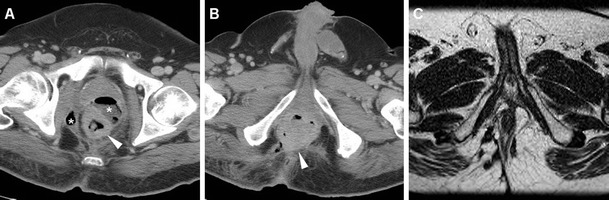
A 53-year-old man with purulent drainage and clinical diagnosis of perianal inflammation. Axial images (b,c) from urgent contrast-enhanced MDCT show solid circumferential thickening of the anal canal (*arrowheads*) associated with abscess collections with mixed gas-fluid content (*) and fistulas crossing the ischioanal space. Surgical examination under anaesthesia including biopsies revealed ulcerated SCAC with superinfection. After surgical drainage and subsequent chemo-radiotherapy, MRI (c) shows complete resolution of both inflammatory and neoplastic changes with residual T2-hypointense fibrotic tracks
